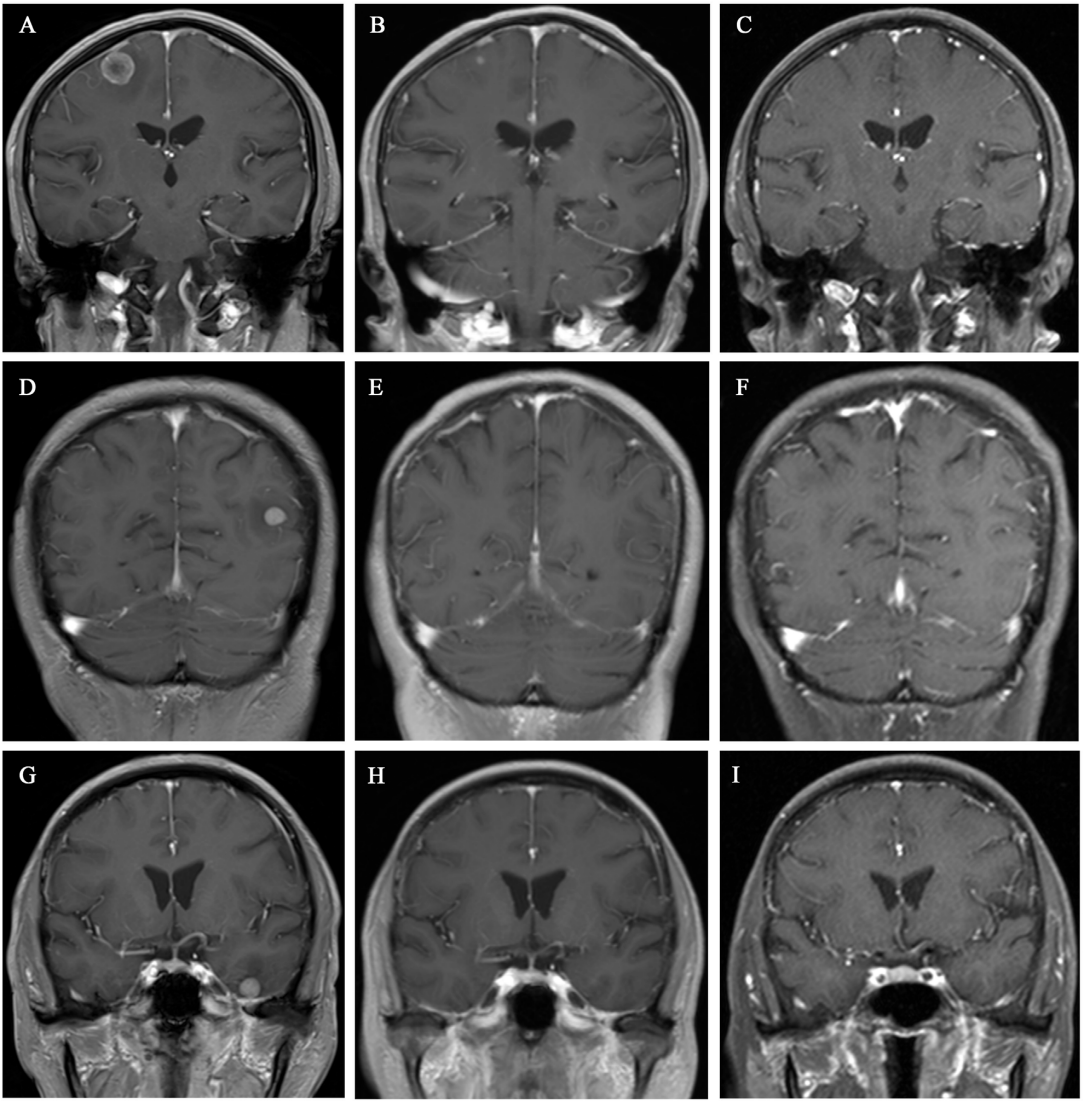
Cranial MRI showed gradual shrinkage of the brain metastases in various regions. (A–C) Coronal images of the left parietal lobe taken on 20 March 2023, 10 July 2023, and 14 November 2023, respectively. (D–F) Coronal images of the right temporal lobe taken on 20 March 2023, 10 July 2023, and 14 November 2023, respectively. (G–I) Coronal images of the right occipital lobe taken on 20 March 2023, 10 July 2023, and 14 November 2023, respectively.
